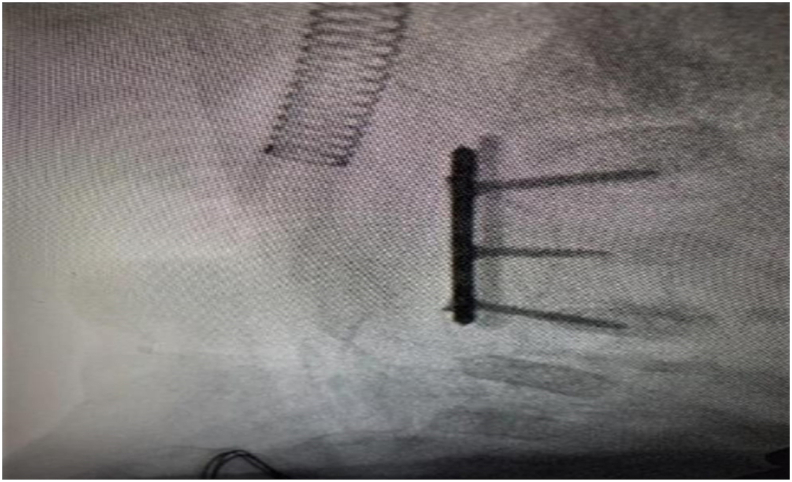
Intraoperative X-ray showing properly placed iliac bone graft after C3 corpectomy and fusion C2–C4 with plate and screws with correction of the kyphotic deformity.
